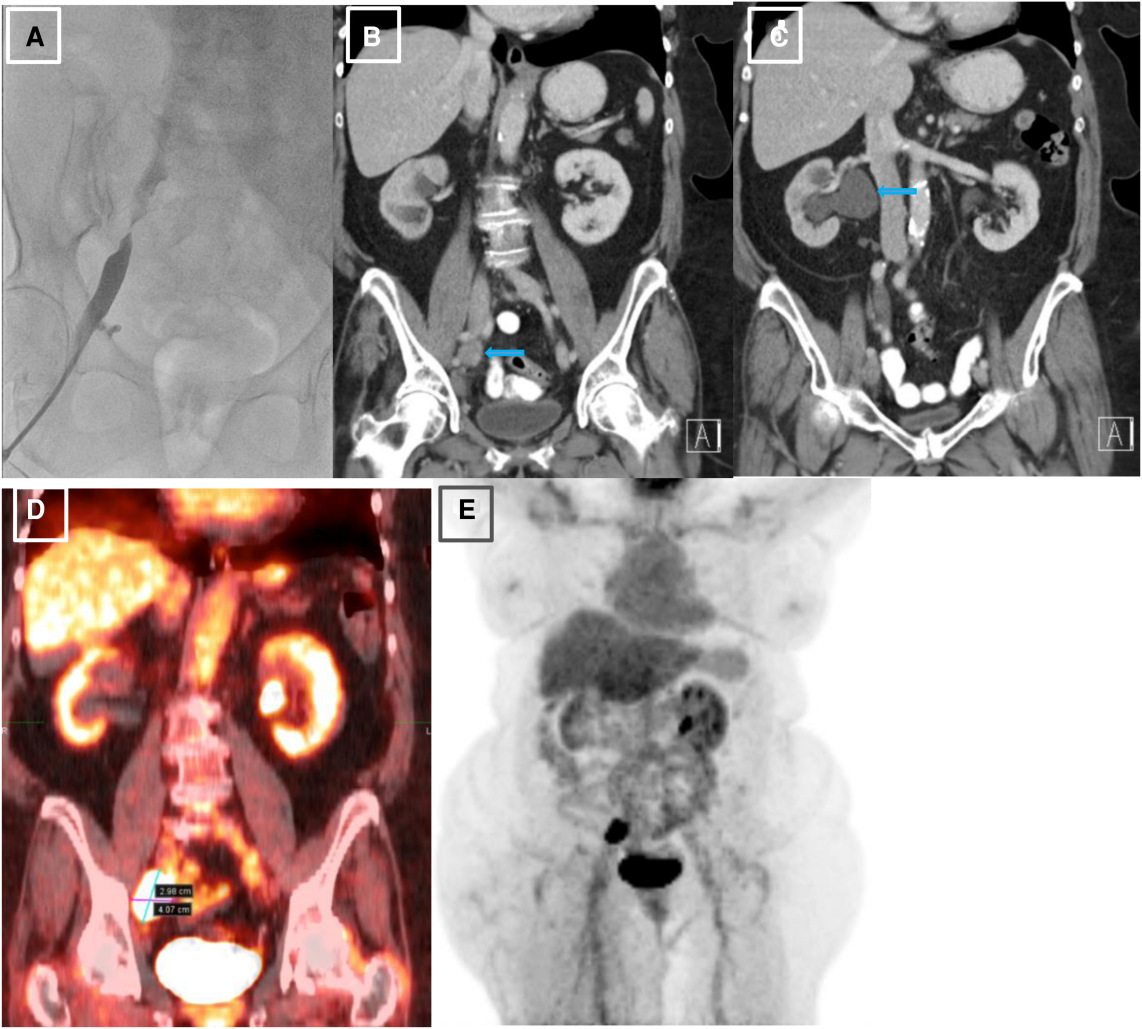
Initial venography, CT and PET imaging of the right external iliac vein. (A). Iliac venography demonstrating severe stenosis of right external iliac vein. (B). CT abdomen pelvis revealing right external iliac lymphadenopathy (LAD), measuring 2.5 × 3.2 × 2.7 cm, marked by blue arrow. (C). CT abdomen pelvis demonstrating severe right sided hydronephrosis due toR ureteral external compression by mass burden, marked by blue arrow. (D, E). PET scan redemonstrating R external iliac LAD.
